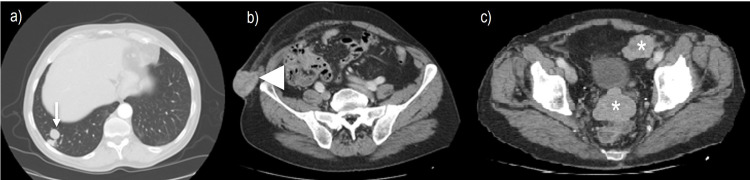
(a) Axial contrast-enhanced CT scan at the level of the thoracic outlet shows metastatic lesions (long arrow). (b) Axial contrast-enhanced CT scan at the pelvic level showing cutaneous metastasis. (c) Axial contrast-enhanced CT scan at the pelvic level showing solid nodules surrounding the spermatic cord (short arrow). CT: computed tomography
